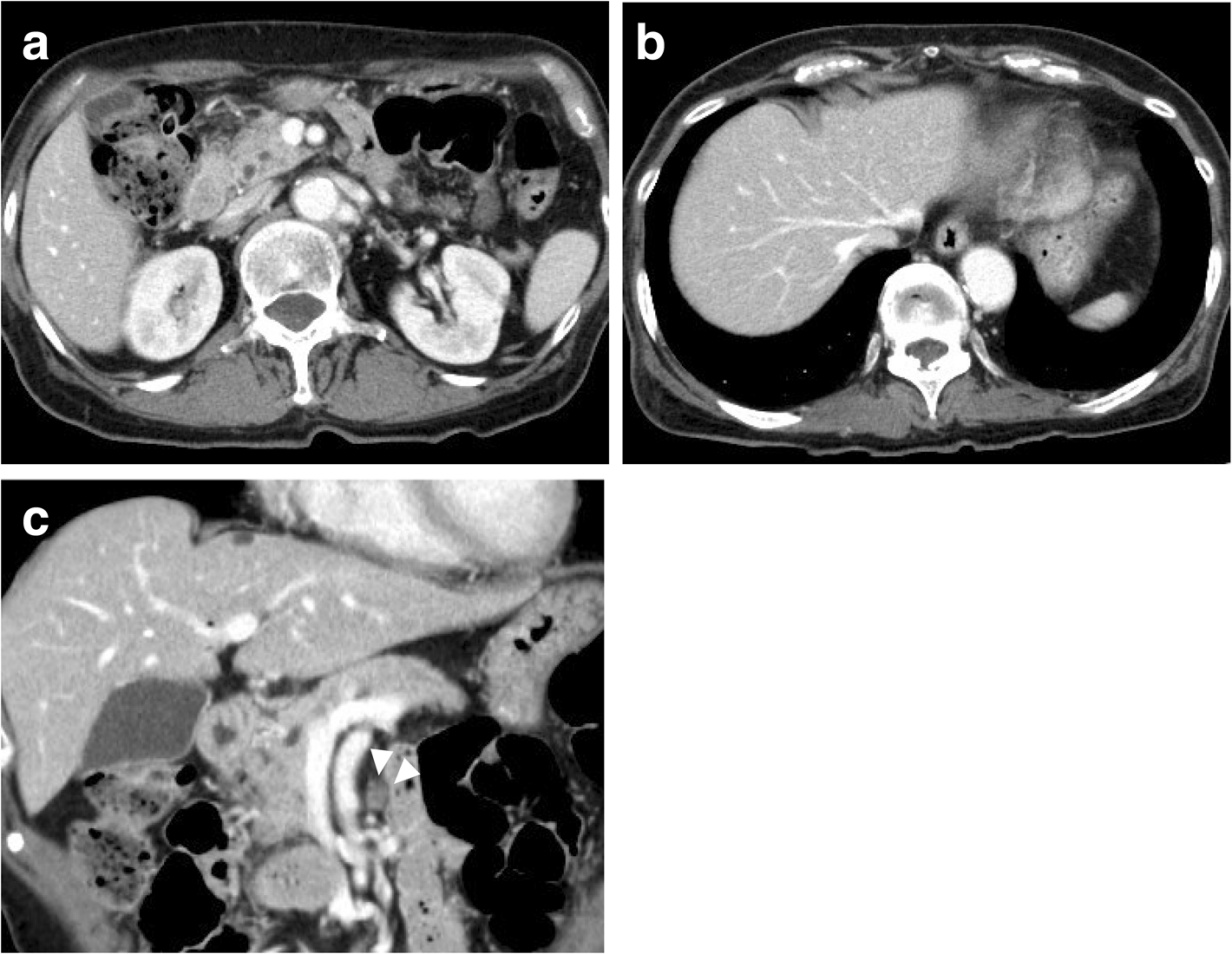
a, b Abdominal computed tomography after 19 months of chemotherapy showed disappearance of multiple liver tumors. c Chemotherapy also contributed to the reduction in the size of the enlarged lymph nodes around the bile duct and superior mesenteric artery (arrowhead)
